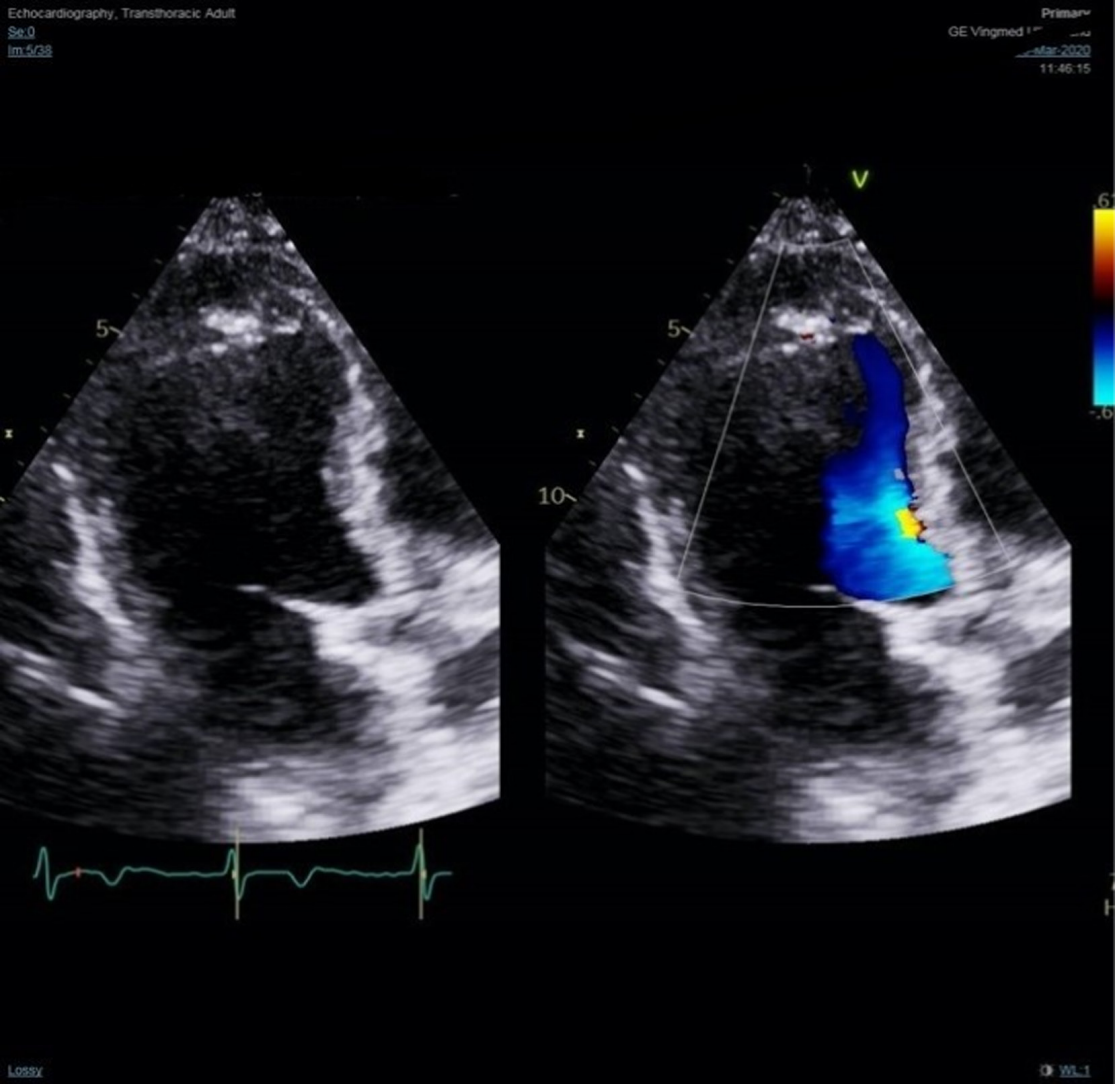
Echocardiography showed apical mass with calcification
